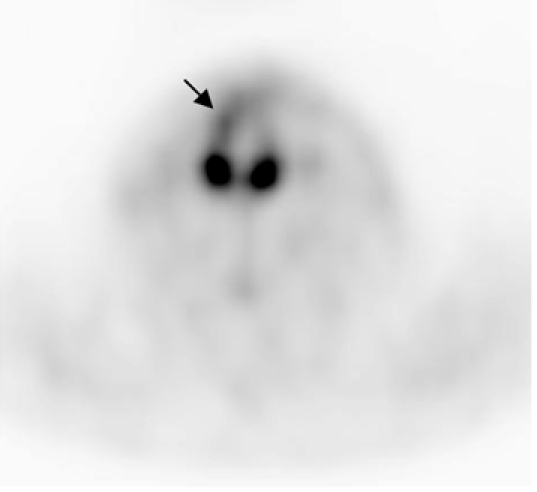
FDG PET scan (axial view) through the larynx. There is asymmetric uptake of tracer seen in the laryngeal muscles while slightly more intense uptake is seen on the right side in this patient with left laryngeal nerve palsy (arrow) [Image courtesy of Centre for Molecular Imaging, Peter MacCallum Cancer Centre, East Melbourne, Victoria, Australia].
